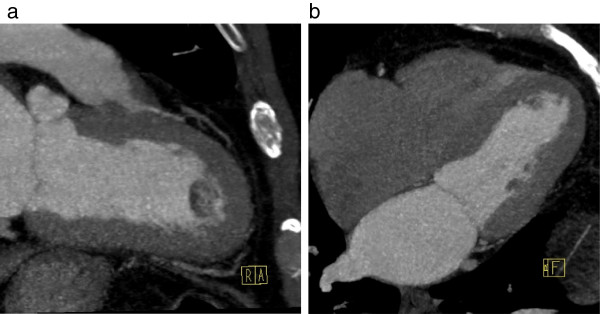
Computed tomography. (a) Two-chamber and (b) four-chamber axial view images revealing a nodular mass at the apical interventricular septum of the left ventricle. R indicates Right, A anterior and F Foot.
